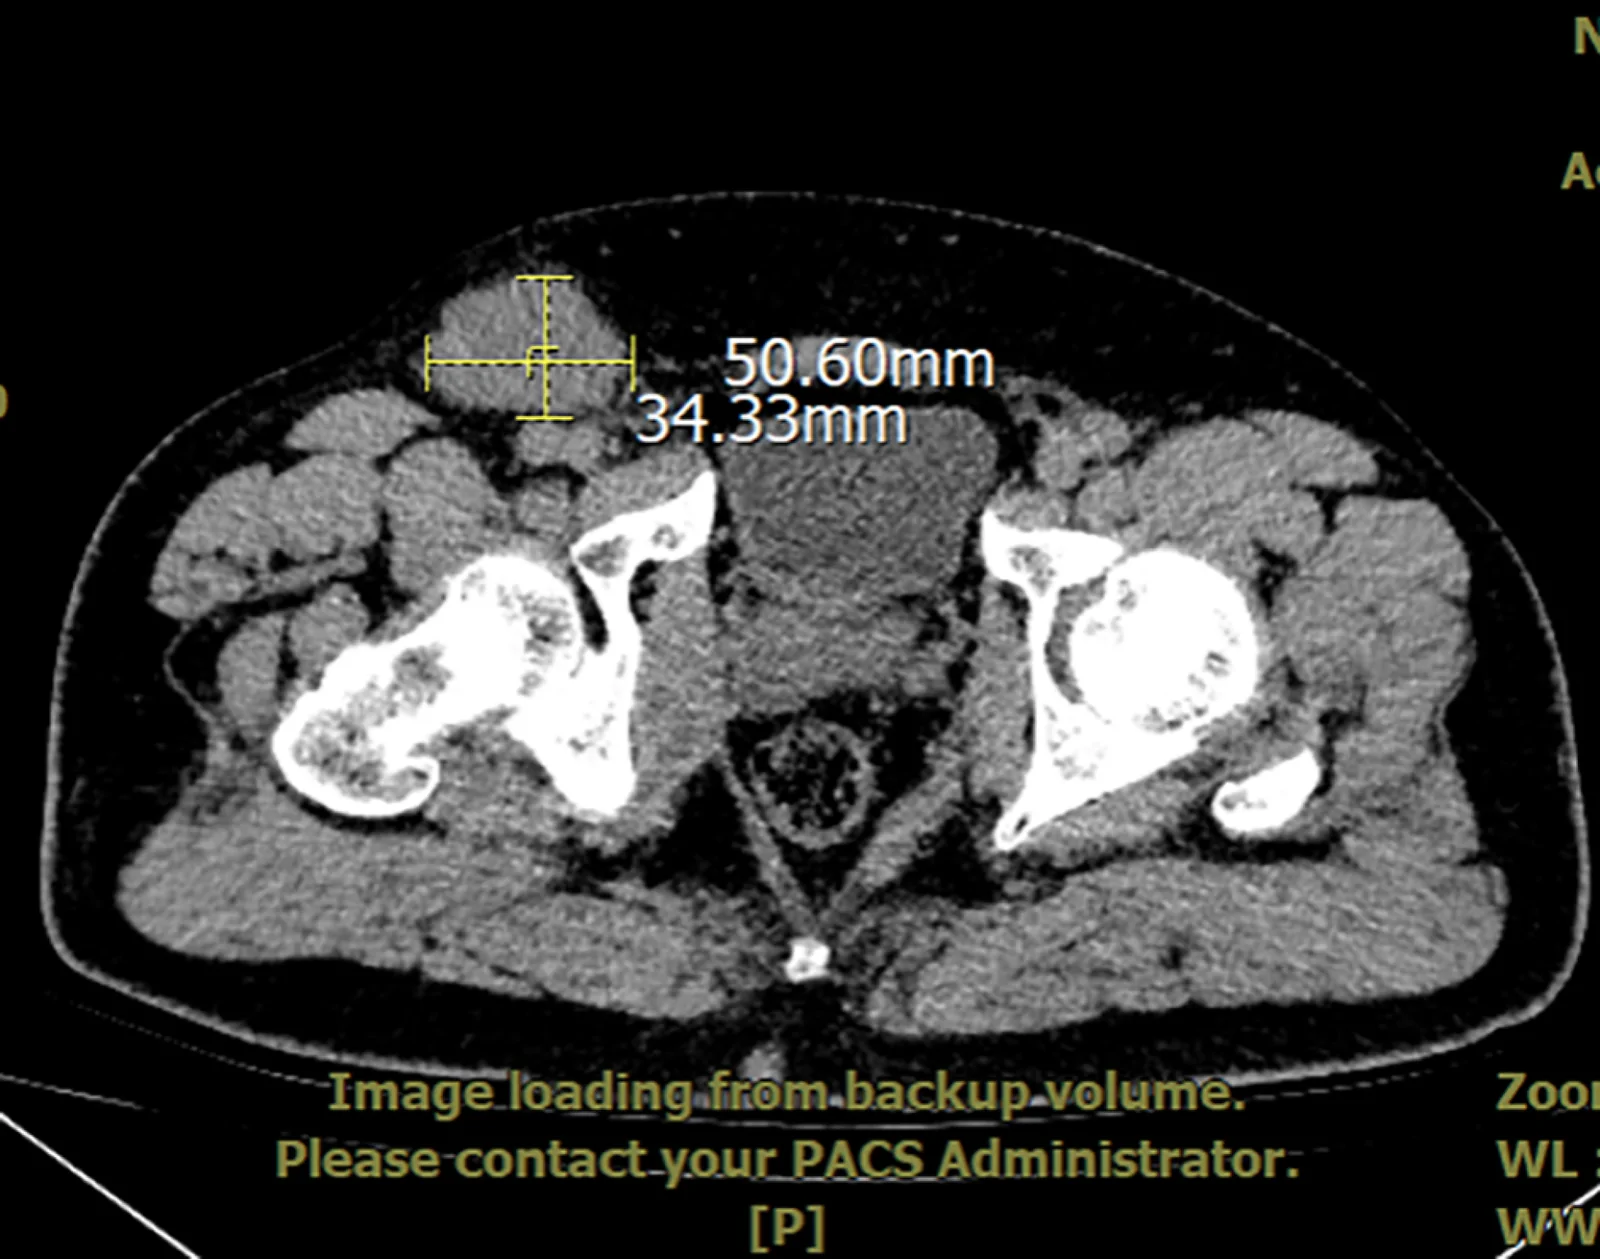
Non-contrast CT scan of the lower abdomen (February 2024) demonstrating a 5.0 cm × 3.4 cm soft tissue mass in the right inguinal region. CT = computed tomography.
